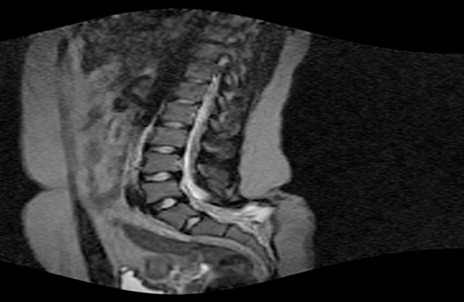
Lumbar MRI showing a lowlying conus medullaris at S1and filum terminale fixed to subcutaneous
